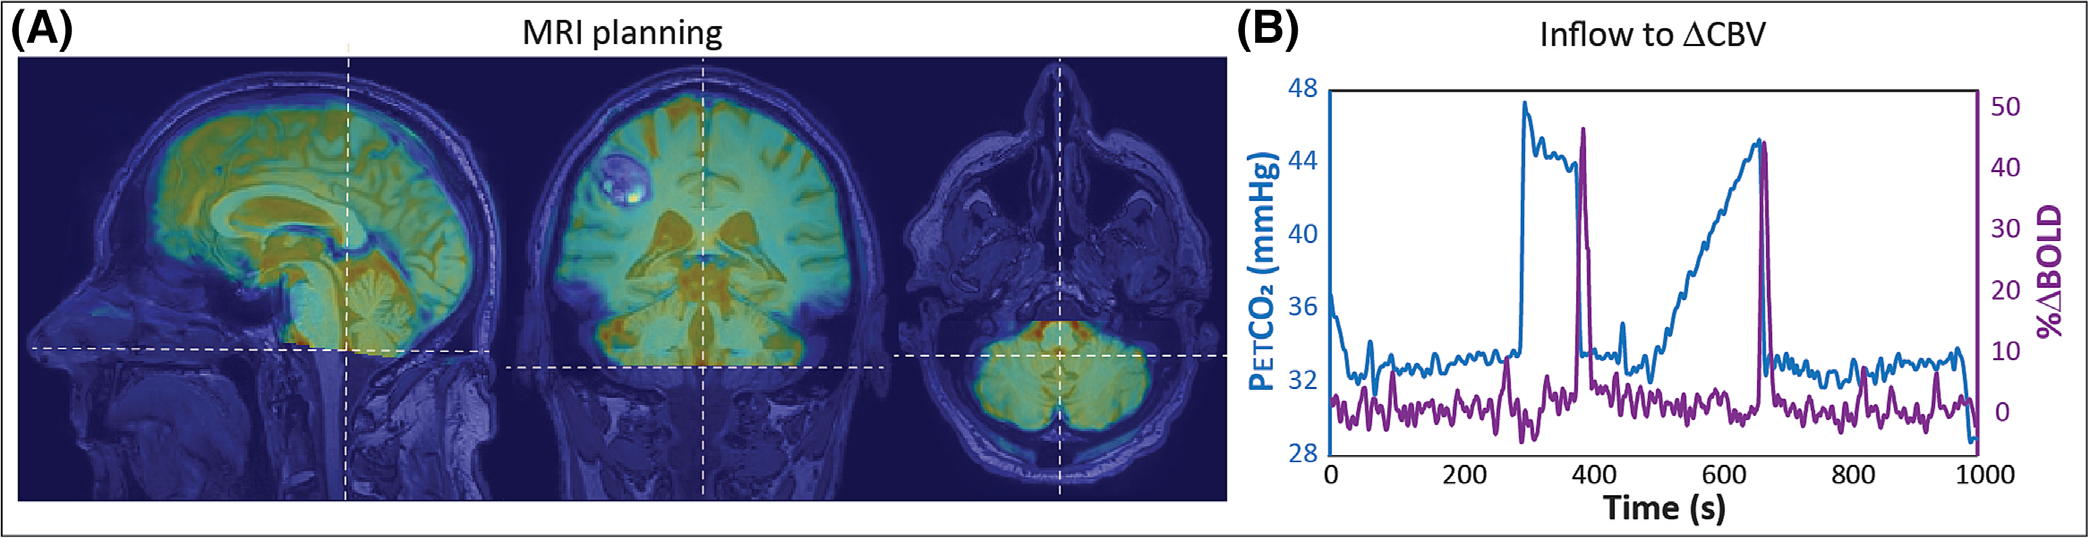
Single subject results: (A) Blood oxygen level-dependent (BOLD) data overlaid on T1-weighted image showing the planning of lower slices just below the fourth ventricle. (B) End-tidal CO_2_ trace (blue) and cerebrospinal fluid inflow signal (purple) as measured in the bottom slices. CBV, cerebral blood volume.
